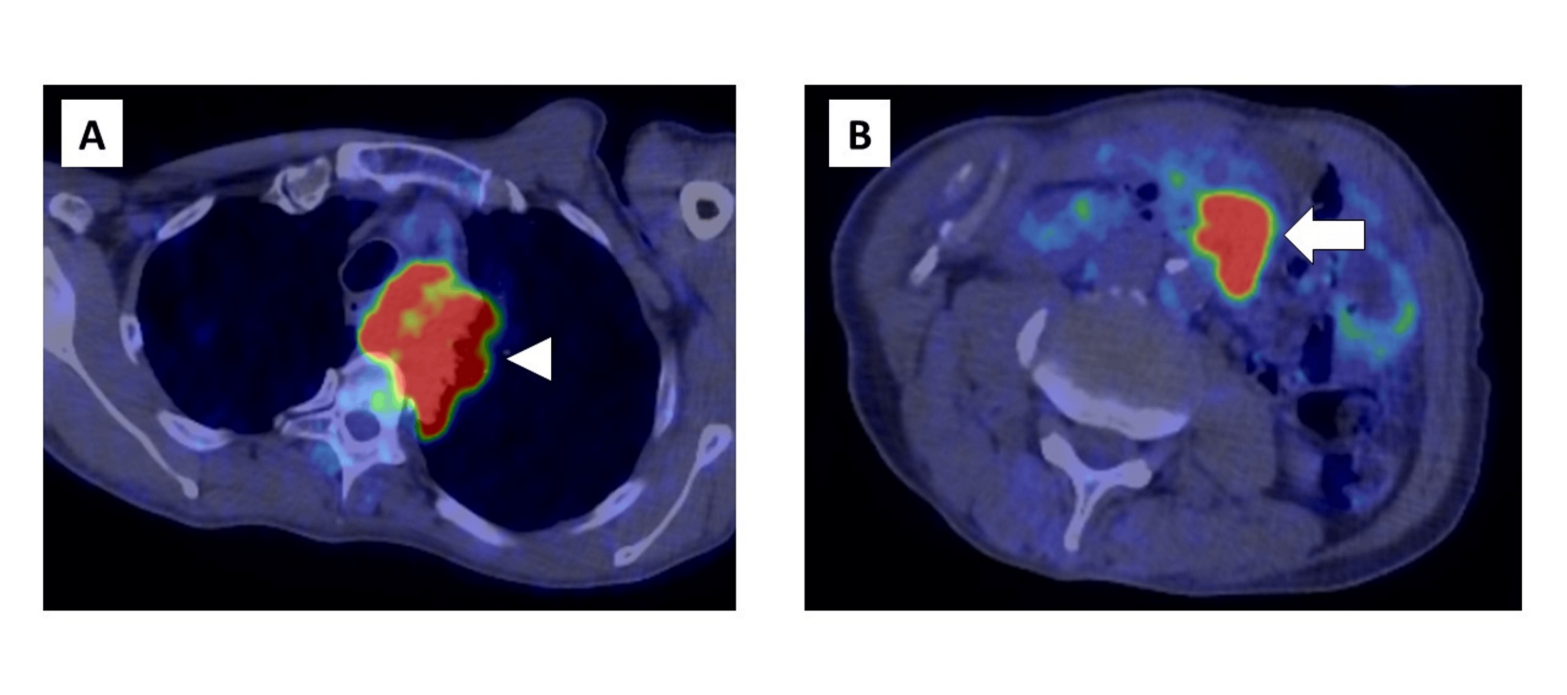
PET findings of chest and abdomen The PET scan showed intense FDG uptake in the mediastinal tumor (arrowhead) and in the small intestinal tumor (arrow). FDG, fluorodeoxyglucose; PET, positron emission tomography
